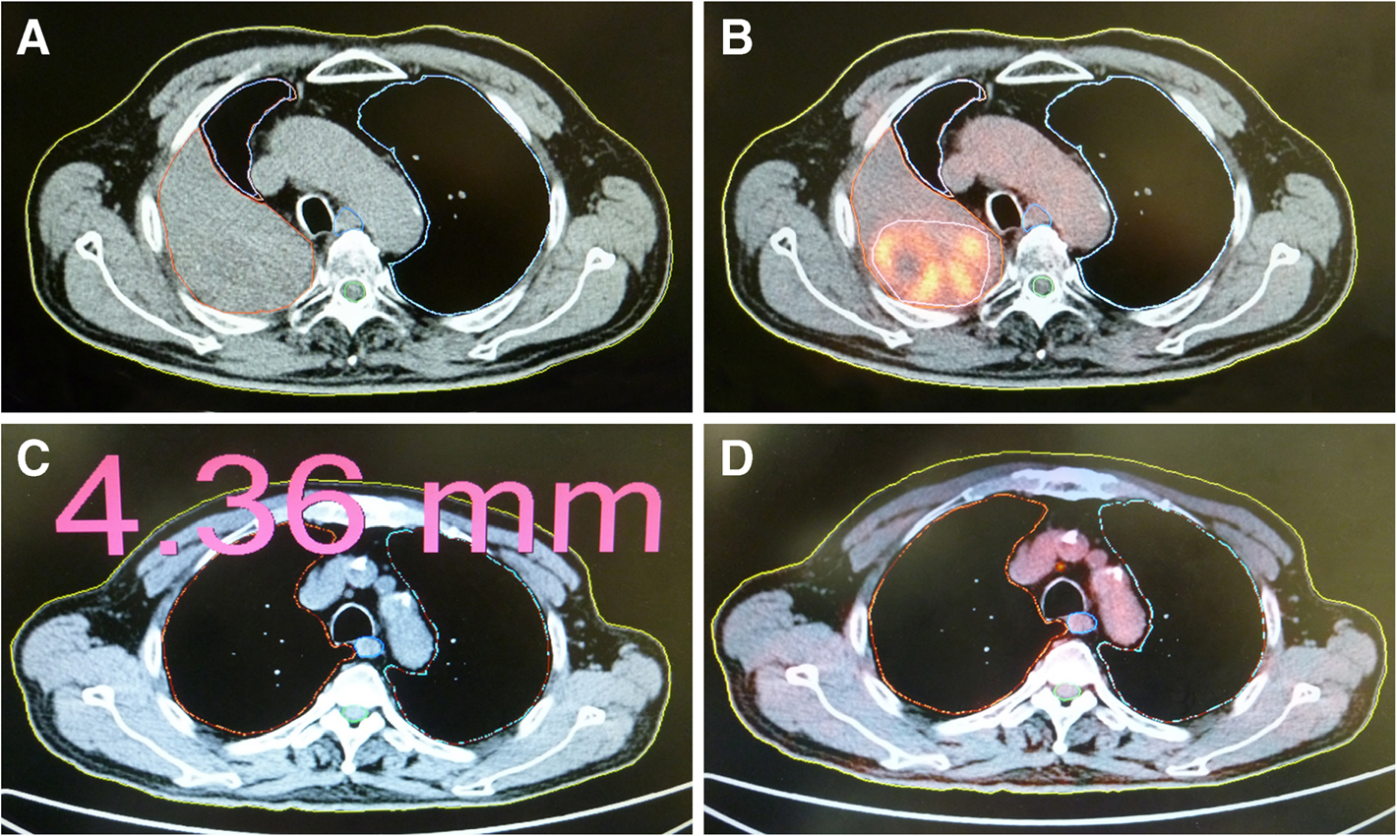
Comparison between conventional CT and PET-CT image. A: By conventional CT, it was difficult to distinguish the boundaries between incompletely expanded lung tissue and tumor tissue with consequent excessive target delineation. B: By PET-CT, the areas of high metabolic activity indicated the presence of tumors. The target volume was obviously smaller than that on conventional CT image. C: On conventional CT image, the mediastinal lymph node had a short-axis diameter of 4.36 mm and was not considered a metastatic lymph node. D: On PET-CT image, the mediastinal lymph node showed high metabolic activity and was considered a metastatic lymph node.
